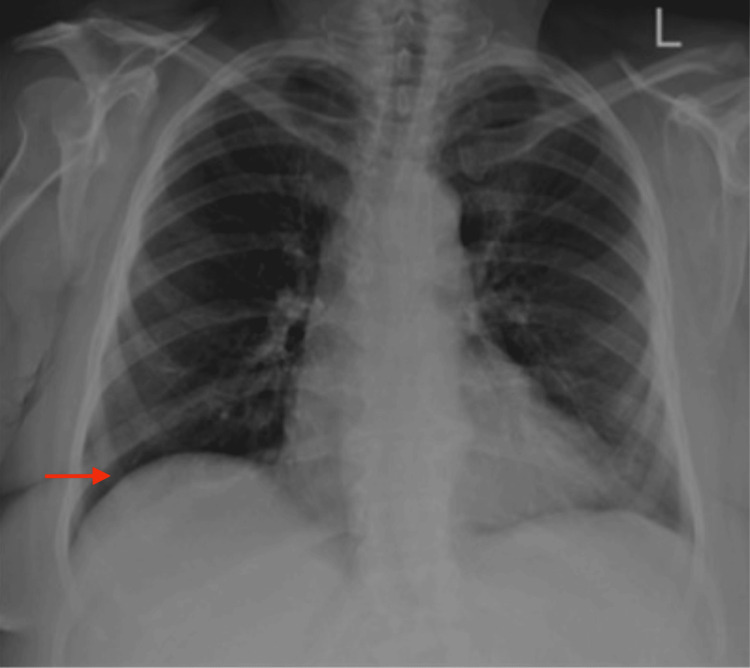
Preoperative Chest Radiograph Preoperative chest radiograph demonstrating no acute cardiopulmonary abnormality. The red arrow highlights a normally positioned right hemidiaphragm.
